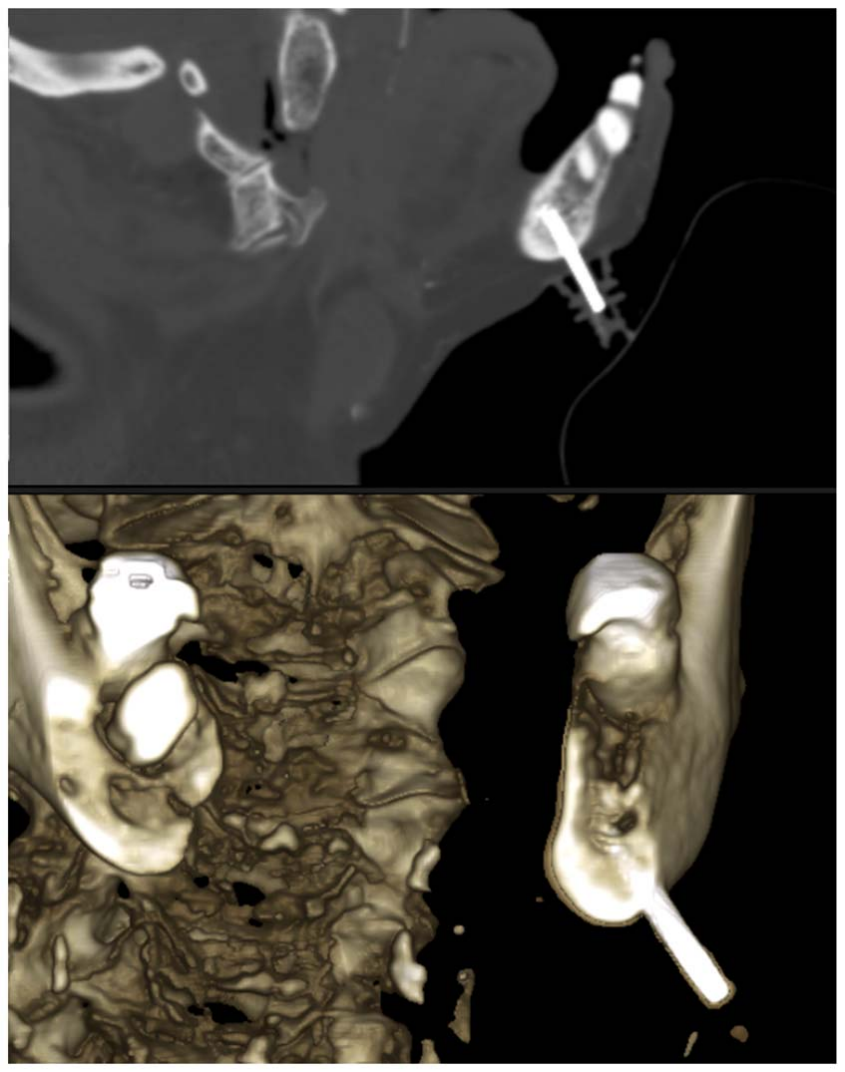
CT scan of a separate experiment is showing a successful puncture ending at the cortical layer of the left anterior mandible.
